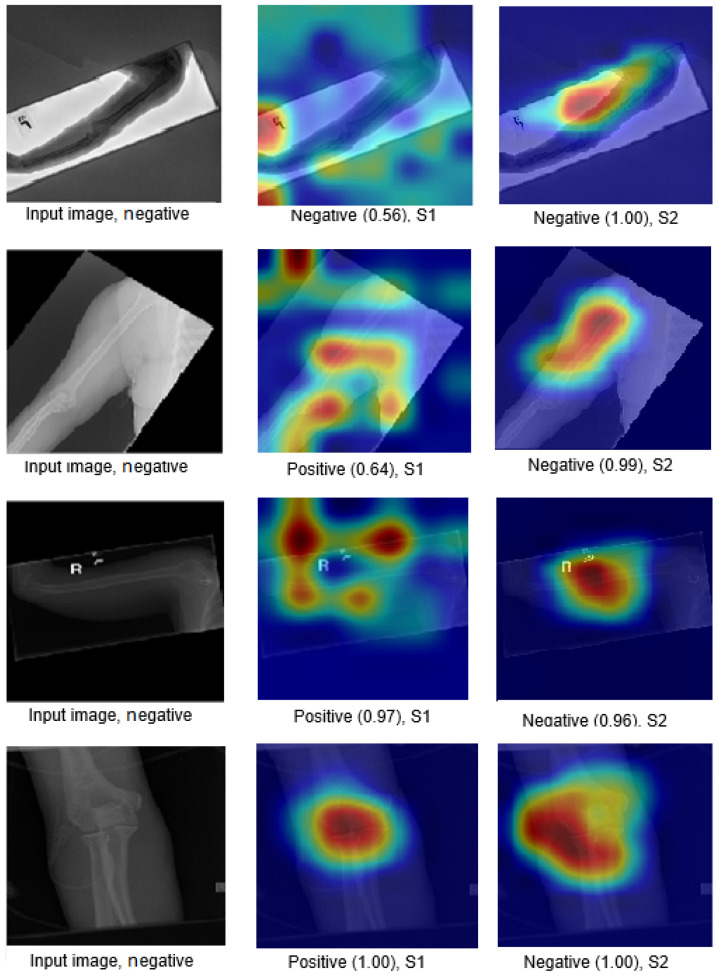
Grad-CAM and score Grad-CAM for the humerus X-ray images. The correct classification is negative.Wrist task: The confusion matrix was calculated for all training cases in both models of DL, and the results are shown in Figure 10 and Figure 11. Using the values of the confusion matrix, various assessment measures were derived. The results demonstrate that S2 outperforms the other model with the Xception DL model, and S2 achieves the highest performance. Specifically, S2 achieves an accuracy of 84.07%, a recall of 73.56%, a precision of 88.93%, a specificity of 92.58%, an F1-score of 80.52%, and a Cohen’s Kappa of 68.11%. In contrast, S1 achieves an accuracy of 69.10%, a recall of 64.29%, a precision of 69.77%, a specificity of 73.65%, an F1-score of 66.91%, and a Cohen’s Kappa of 38.02%.As in the InceptionresNetV2 DL model, the results demonstrate that S2 achieves an accuracy of 82.85%, a recall of 67.80%, a precision of 91.74%, a specificity of 95.05%, an F1-score of 77.97%, and a Cohen’s Kappa of 64.45%. S1 achieves an accuracy, recall, and precision of 80.21%, 74.29%, and 83.81%, respectively. Meanwhile, the different performance metrics obtain a specificity of 85.81%, an F1-score of 78.49%, and a Cohen’s Kappa of 60.27%.These results highlight the superior performance of S2 compared to S1. S2 shows superior precision, recall, specificity, and F1-score, highlighting its classification ability. The higher Cohen’s Kappa value suggests better agreement between the predicted and true labels. On the contrary, S1 exhibits lower performance in all assessment measures, indicating its limitations in accurately classifying data.
